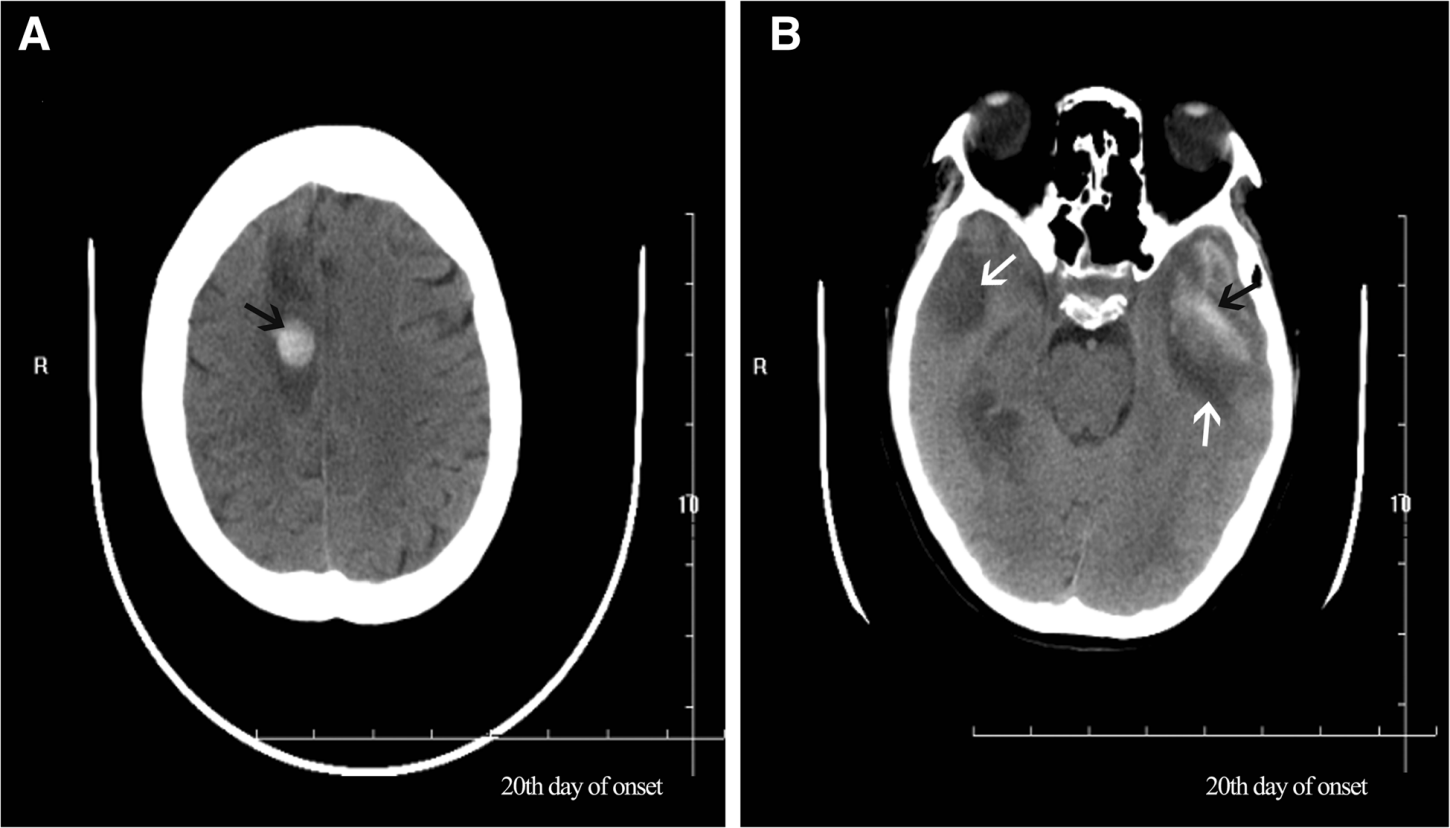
a CT scan of the brain showed multiple hypodense lesions (white arrows) in the right frontal lobe, right insula, bilateral temporal lobe and left basal ganglia, including right frontal lesion hemorrhage (black arrow). b CT scan of the brain showed the range of hypodense lesions in bilateral temporal lobes (white arrows) had increased, and hemorrhage within the left lower temporal lobe hypodense lesion (black arrow)
